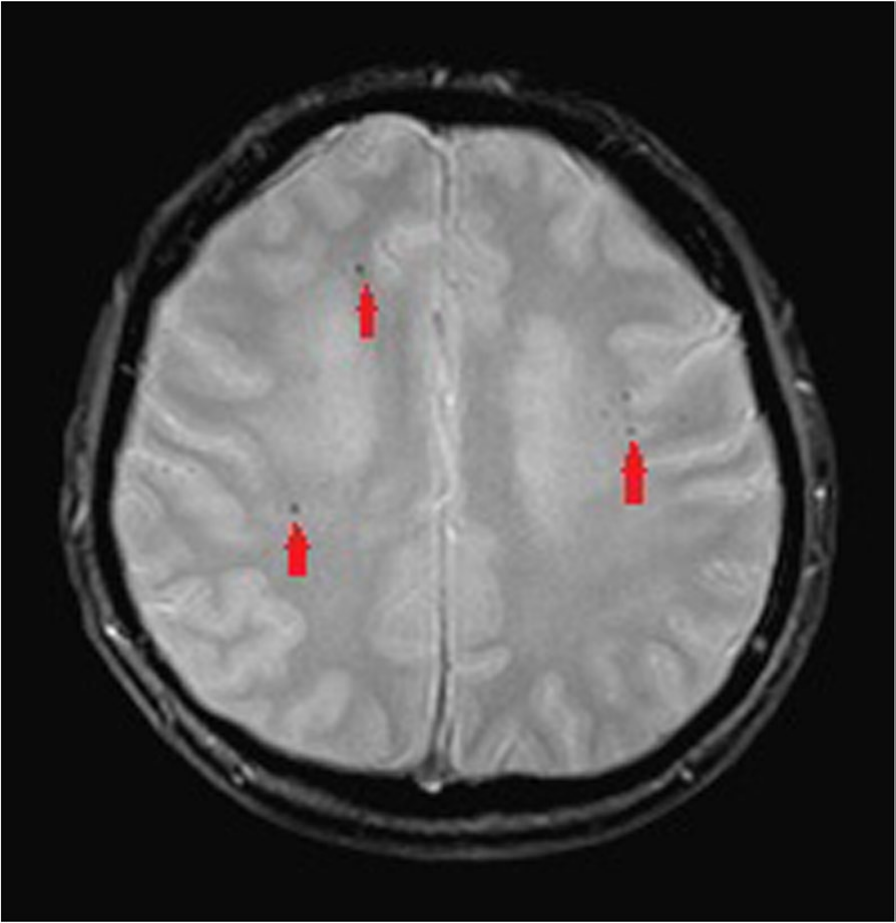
The hypointensity on the Axi MPGR (MPGR = multiplanar gradient-recalled) series on brain MRI indicates multiple small old ICH in multiple areas, leading to a strong suspicion of hypertensive microangiopathy ICH. (Red arrows)
